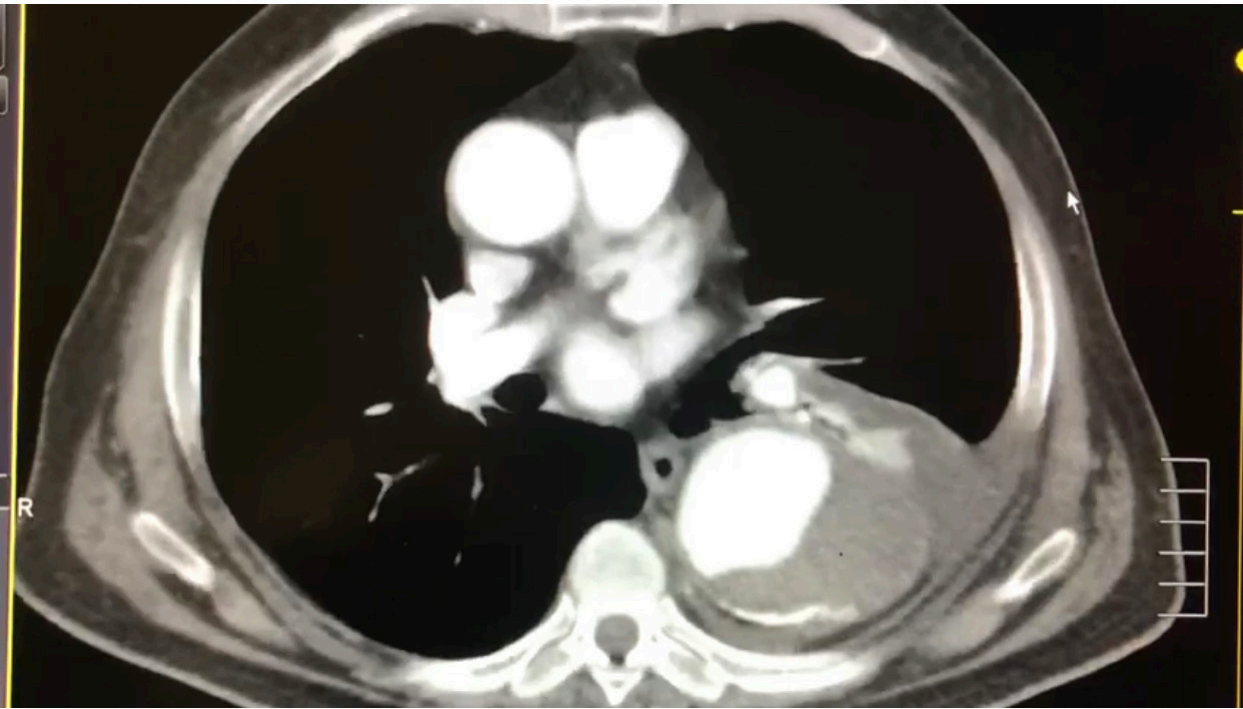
Incidental cardiovascular findings in computed tomography of the chest with contrast. Descending thoracic aortic aneurysm.
